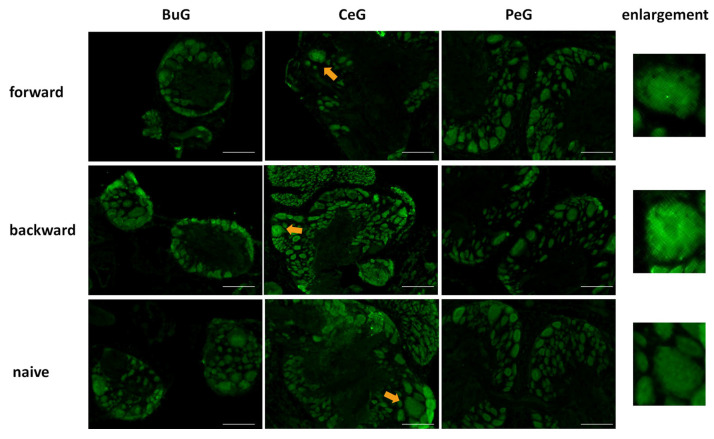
LymFOXO localization changes in CNS sections of food-deprived Day 1 snails after CTA conditioning. BuG: buccal ganglion. CeG: cerebral ganglion. PeG: pedal ganglion. Green indicates the immunoreactivity of LymFOXO. Arrows indicate enlarged neurons shown in the right panels. The enlarged figure of the CNS of a forward-conditioned snail shows that LymFOXO is present in the nucleus. Scale bars: 100 µm.
